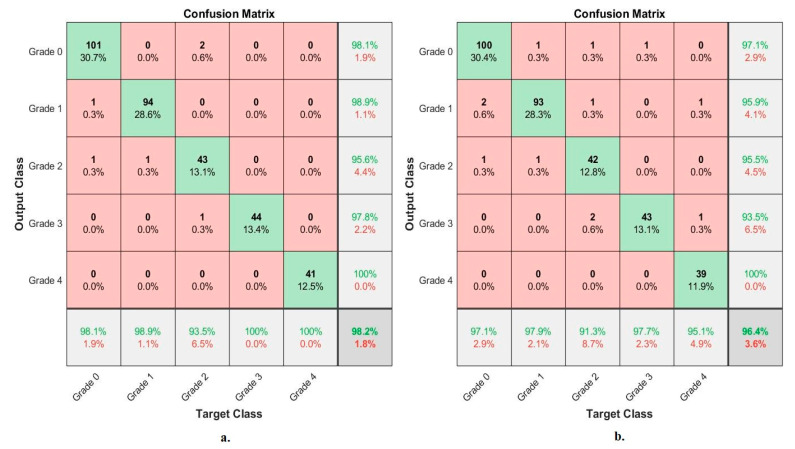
Display of the confusion matrix of the X-ray analysis of an RCU dataset for early diagnosis of severe KOA by FFNN with features of (a) VGG-19-PCA-handcrafted (b) ResNet-101-PCA-handcrafted.
